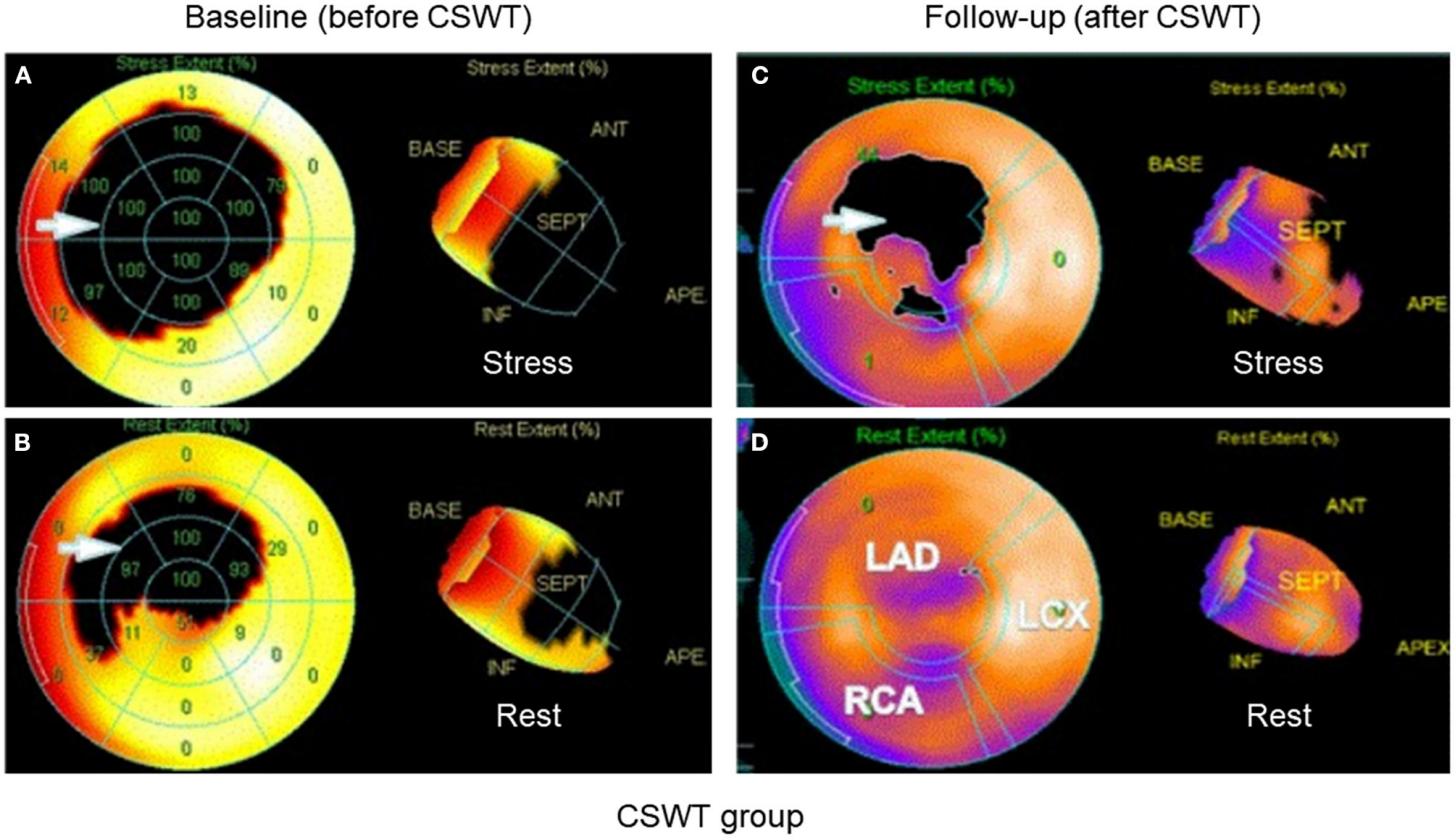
D-SPECT of a patient. After CSWT, the ischemic myocardium at rest and stress procedure was significantly reduced at 6 months after treatment as compared to that before CSWT. (A) Before treatment at stress procedure. (B) Before treatment at rest procedure. (C) After treatment at stress procedure. (D) After treatment at rest procedure. Arrow: ischemic area. CSWT, cardiac shock wave therapy; D-SPECT, dynamic single photon emission computed tomography; LAD, left anterior descending artery; LCX, left circumflex coronary artery; RCA, right coronary artery.
